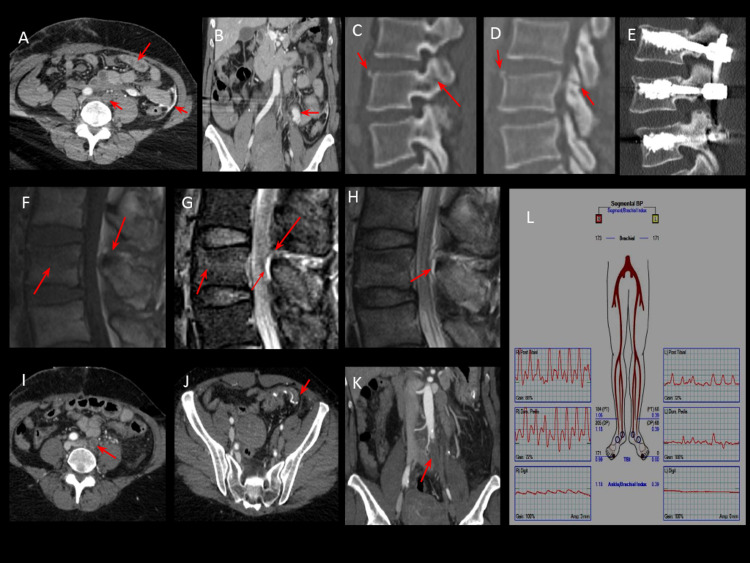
Acute occlusion of the left common iliac artery (A, B, I, J, and K) CT demonstrating occluded left common iliac artery, sigmoid colon injury with active extravasation, hemoperitoneum, and small bowel edema. (C, D, E) CT demonstrating unstable chance fracture status post-posterior percutaneous fusion with vertebroplasty. (F, G, H) MRI of the lumbar spine demonstrating unstable chance fracture with small epidural hematoma. (L) Ankle-brachial index of the patient demonstrating severely decreased ratio of 0.39 in the left posterior tibial artery and 0 in the dorsalis pedis artery, indicating severe arterial insufficiency compared to normal 1.1 on the right side.
